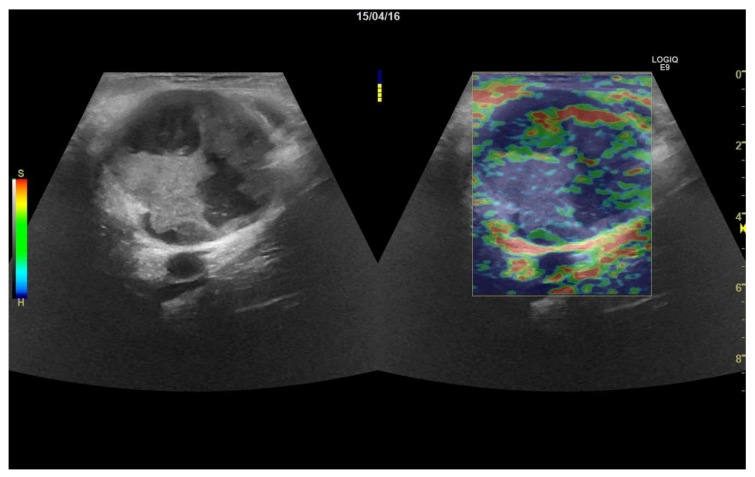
A 74-year-old female with metastasis of malignant melanoma. Ultrasound shows a highly heterogenous tumor, with both soft (red) and hard (blue) areas. The tumor was given a TES of 4 and a SR of 1.44.
